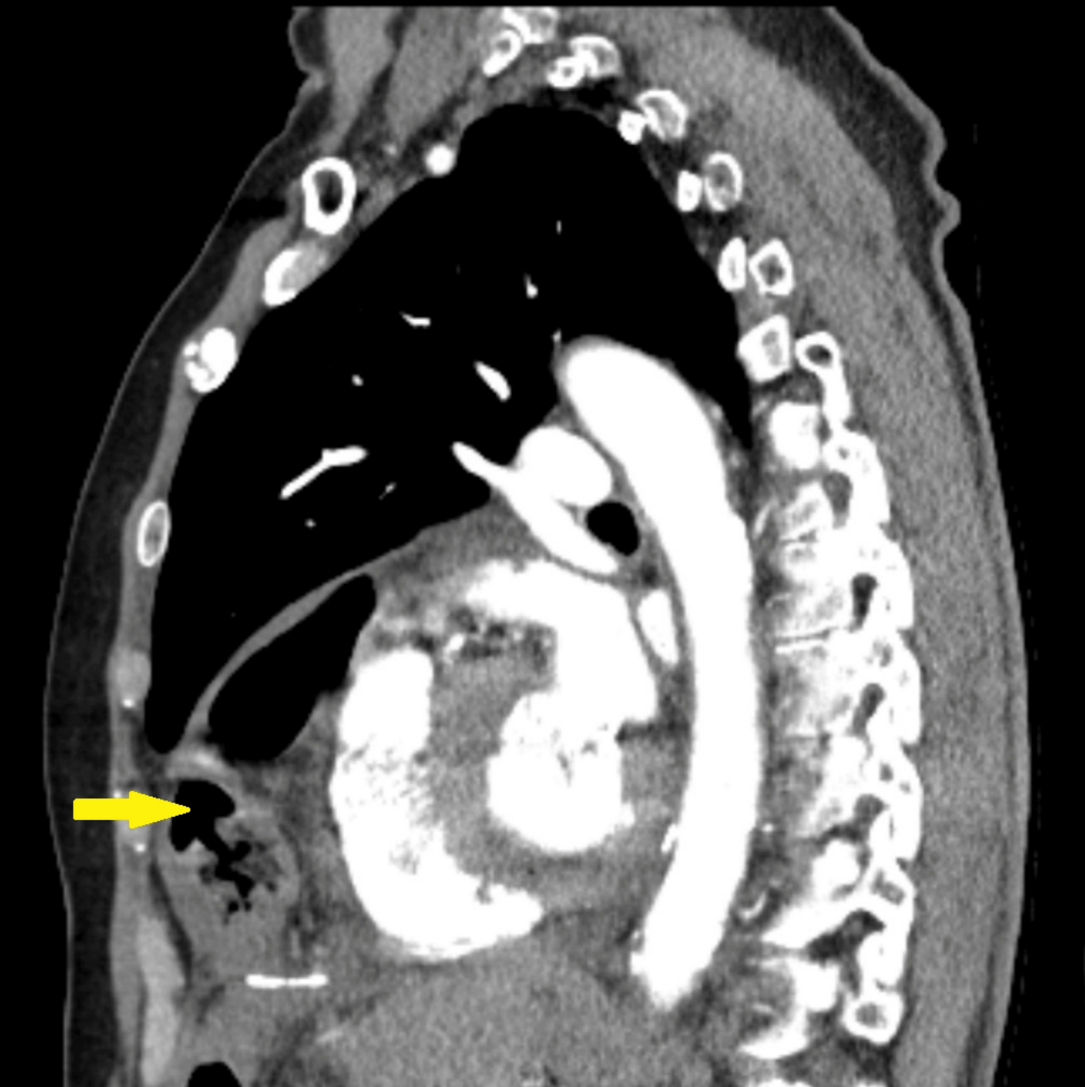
CT sagittal view showing transverse colon (yellow arrow) anterior to the heart within the pericardial sac
